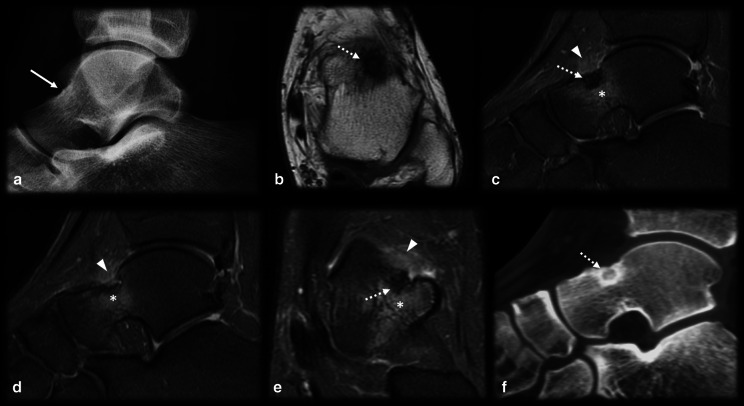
Osteoid osteoma mimicking anterior impingement. A 29-year-old male presenting with anterior ankle pain for 4 months. CR (a) showed mild sclerosis on the dorsal talar neck (arrow). T1 (b), T2 (c, d, e) and CT (f) showed a mineralized nidus (dotted arrows) with reactional synovitis (arrowhead), bone marrow edema (asterisk) and sclerosis
